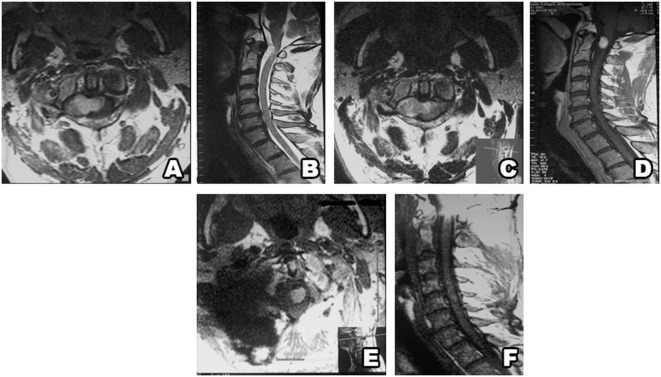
Type II schwannoma (intradural dumbbell-shaped tumor with extension to the extraforaminal region) (A,B), and with contrast enhancement (C,D). Postsurgery MRI confirms gross total resection, without hematoma or recurrence (E,F).
